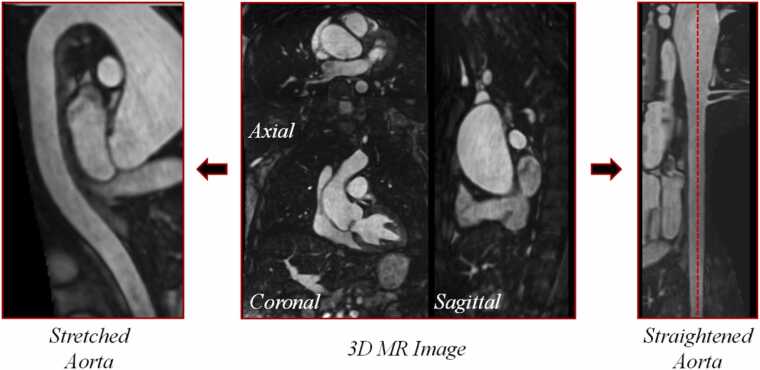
Outcome of (automatic) curved planar reformatting of the aorta. At the center of the image, we see the 3D MR image taken as an example of automatic CPR. On the left, there is the stretched aorta and, on the right, the straightened aorta. *3D* three-dimensional, *CPR* curved planar reformat, *MR* magnetic resonance
